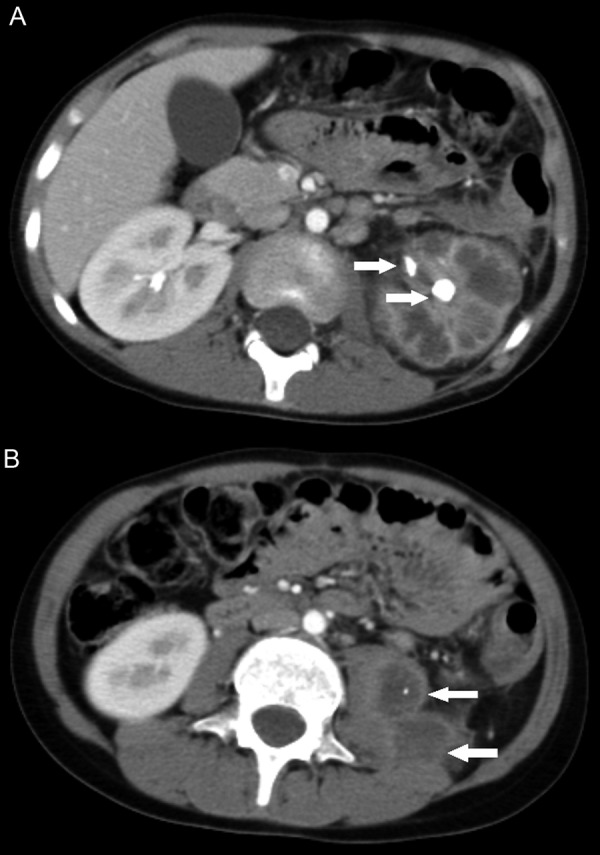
Axial CT source images. A: At the level of the renal hila. The swollen left kidney enhances poorly following intravenous contrast administration. There is calyceal distension and overlying cortical thinning. High attenuation calculus material is seen in the renal pelvis (white arrows). There is inflammatory stranding in the pararenal fat. B: Just inferior to the left kidney. Well-defined, low attenuation lesions are seen in both the enlarged left psoas and iliacus muscles (white arrows); the former contains a fleck of calcification. The rim of both lesions enhances with the administered intravenous contrast. Appearances are consistent with left-sided xanthogranulomatous pyelonephritis and secondary abscess formation. The psoas abscess contains an extruded calculus.
